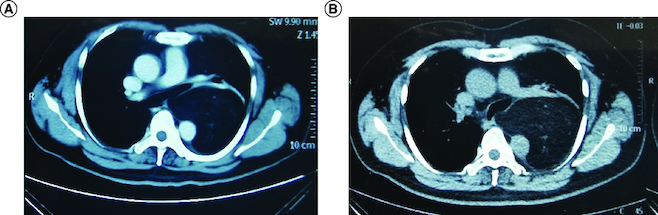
CT images of the tumor. CT picture before first surgery (A) and recurrence CT picture after first surgery (B).
